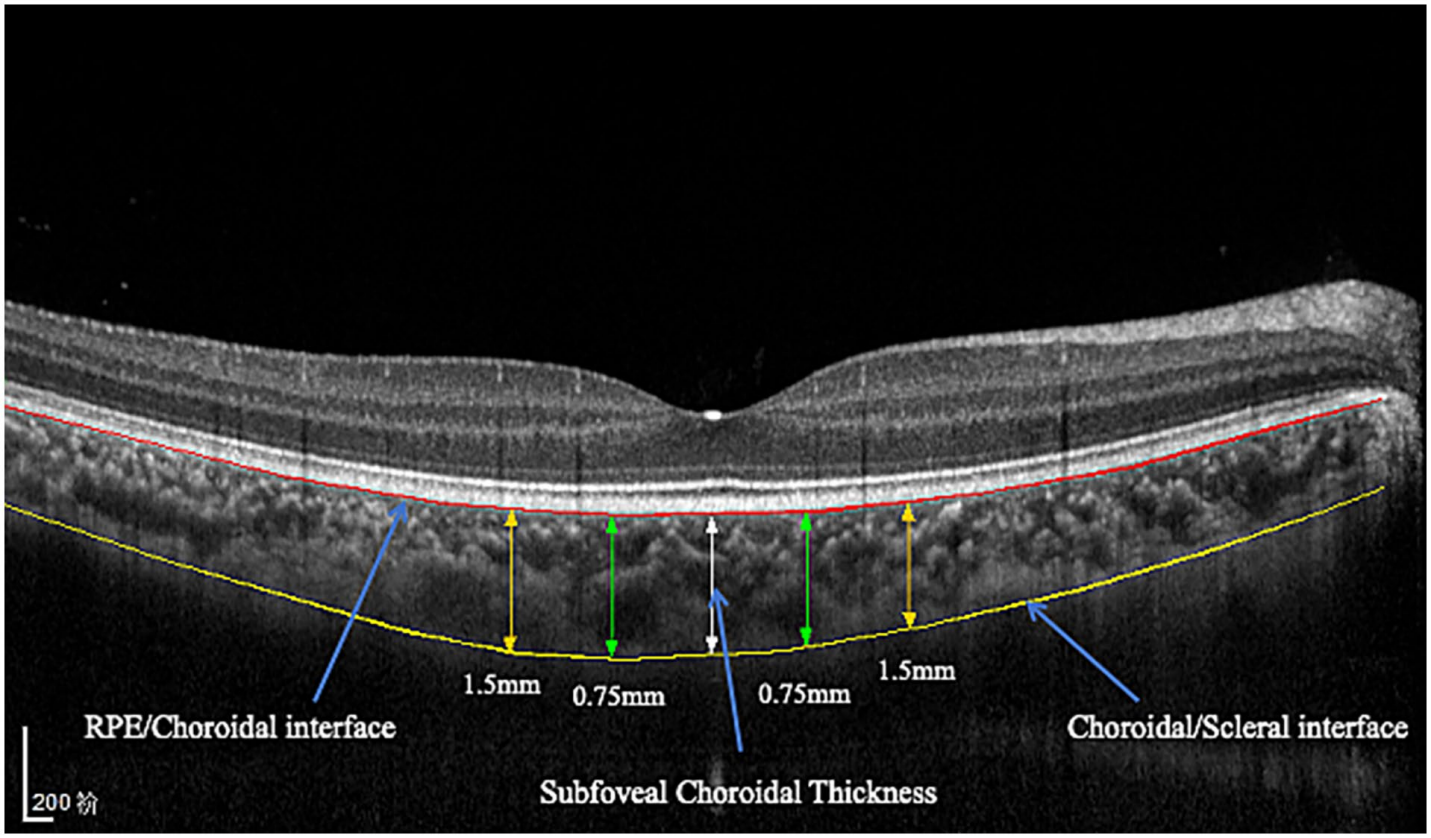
CT measurements on SD-OCT in EDI mode.
